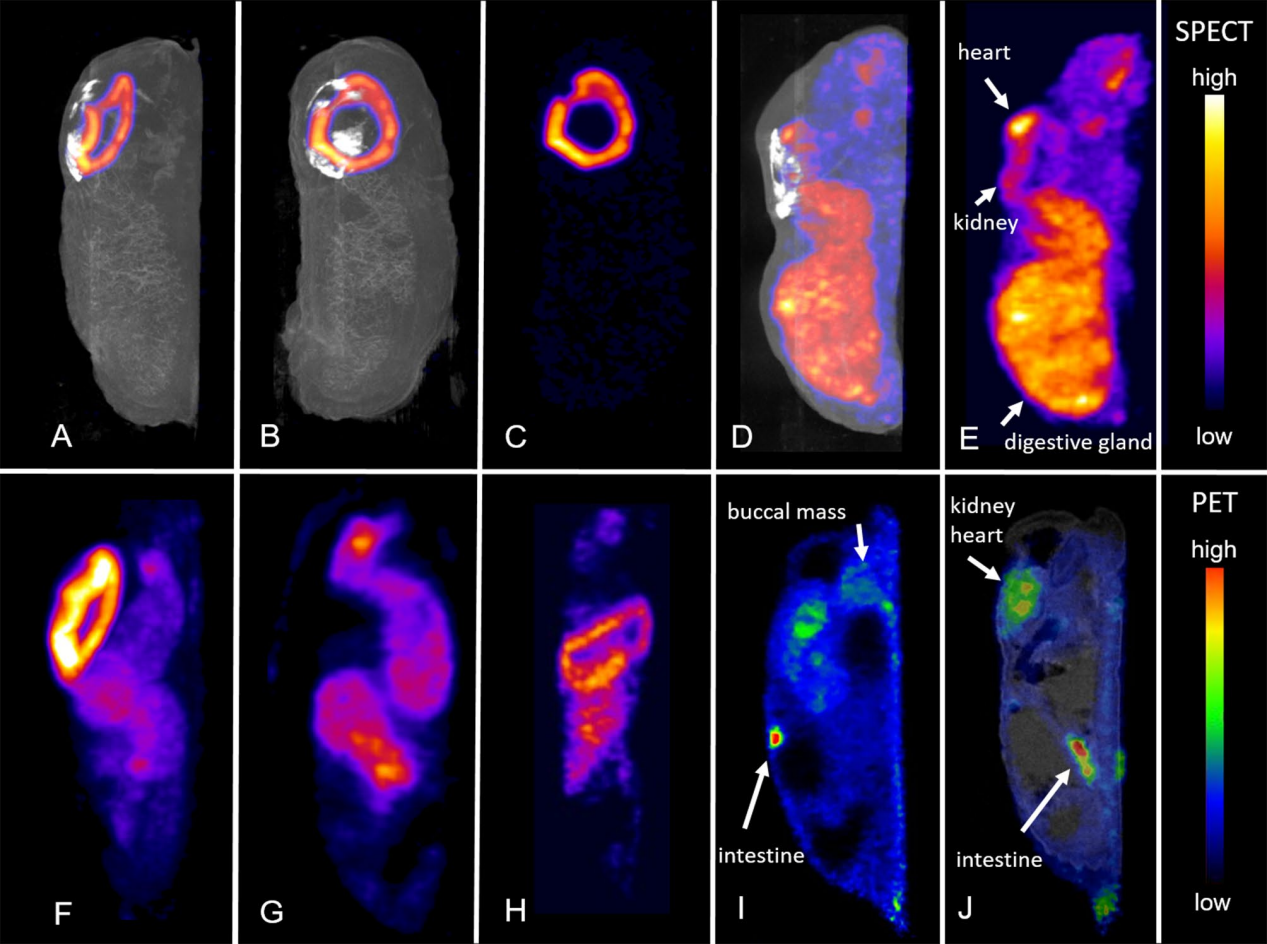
European round back slug (*Arionidae*); Radionuclide images. SPECT images after tracer injection (A–H, maximum intensity projections): ^99m^Tc-MAG3 uptake in the kidney in lateral (A) and dorsal view (B) fused with CT, and SPECT image alone (C). ^99m^Tc-MIBI uptake images in lateral views fused with CT (D) and alone (E). ^99m^Tc-MIBI uptake foci are located in the heart, kidney and digestive gland. SPECT images showing ^99m^TcO_4_^−^ uptake in the kidney (F, lateral view), crop and albumen gland in coronal projections (G, H). Sagittal ^18^F-FDG PET image alone (I) and fused with MRI (J).
